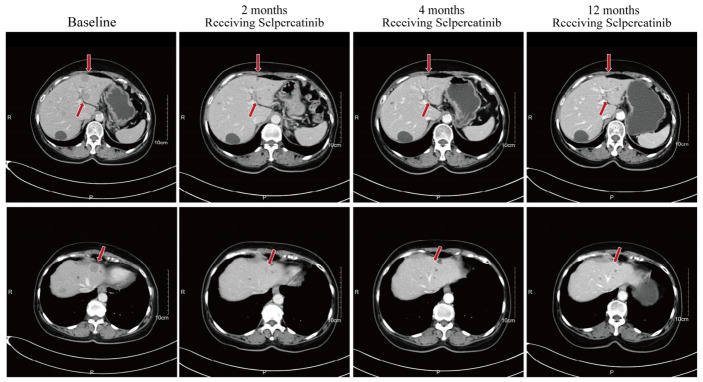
Serial contrast-enhanced abdominal computed tomography showing partial response of hepatic metastases during selpercatinib treatment. Representative axial CT images obtained at baseline and during follow-up after selpercatinib initiation demonstrate a marked reduction in the size of hepatic metastatic lesions over time, consistent with partial response according to RECIST version 1.1. Red arrows indicate representative hepatic target lesions. The upper and lower rows show two representative axial levels. Abbreviations: CT, computed tomography; PR, partial response; RECIST, Response Evaluation Criteria in Solid Tumors.
